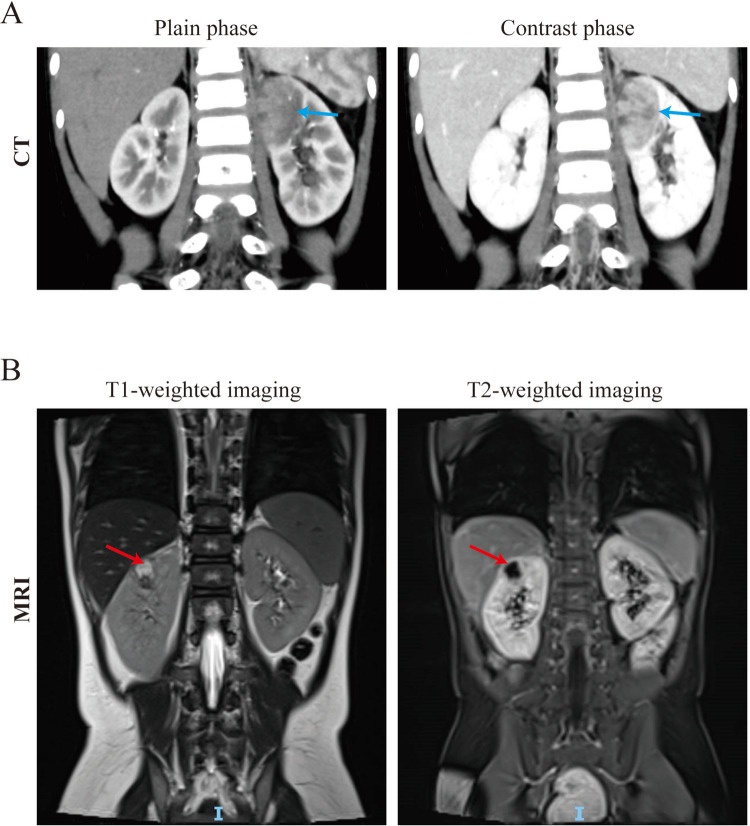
Size of renal abscess and related clinical data. (A) Imaging features of renal abscesses on plain and contrast-enhanced CT, with blue arrows marking CT changes. (B) MRI images highlighting abscesses with red arrows
